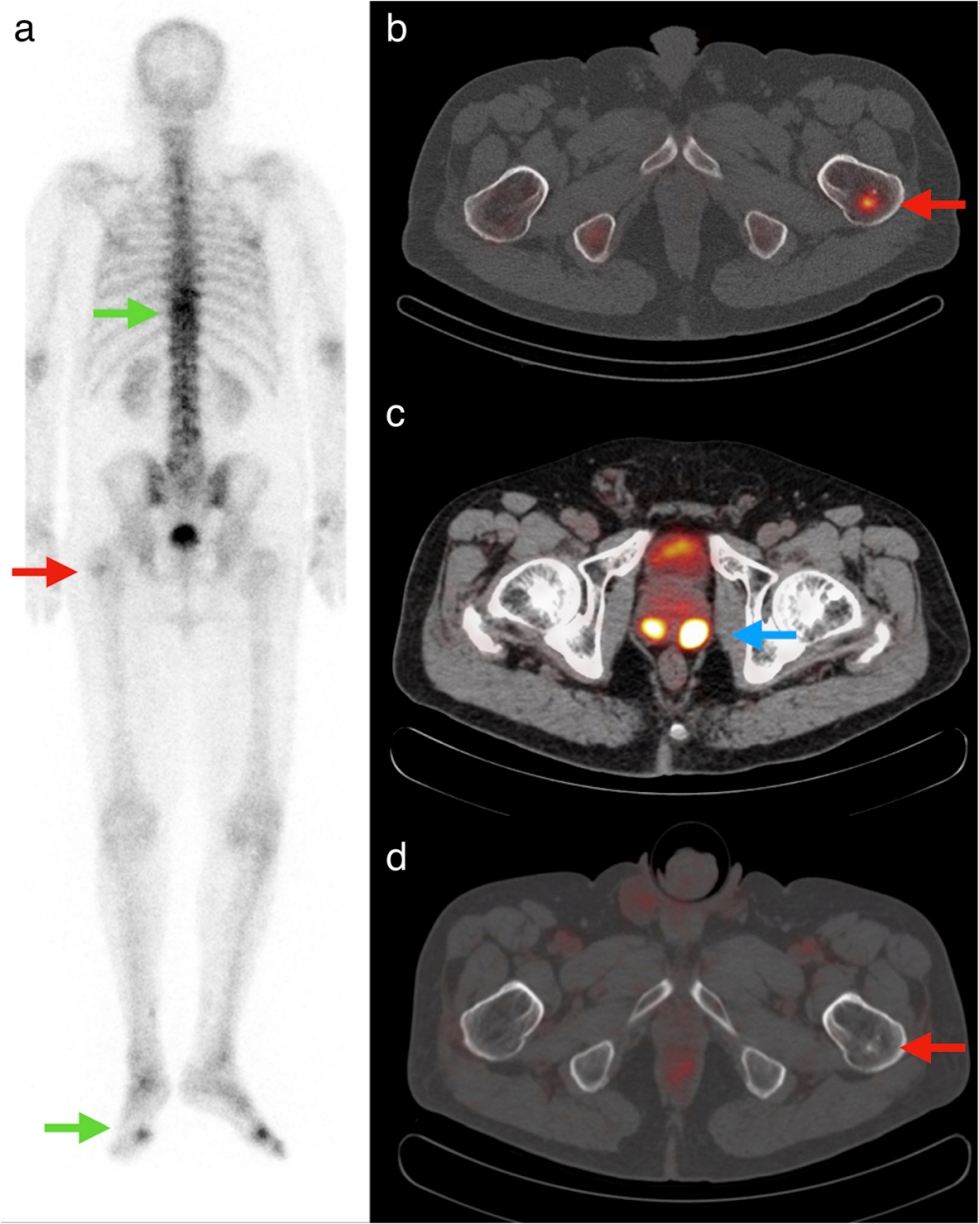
Example of staging change of PSMA PET/CT compared with conventional imaging. A man presented with a clinical T2c Gleason 7b (predominant Gleason score 4) PCa with an iPSA of 16. Planar BS (a) showed a faint spot in the left proximal femur (red arrow) which was confirmed on SPECT/CT (b) as a sclerotic lesion with osteoblastic activity (red arrow). The lesion was interpreted as suspicious for bone metastasis. Other areas with increased osteoblastic activity (green arrows) were interpreted as degenerative. A [^68^Ga]PSMA PET/CT (c) 36 days later shows a PSMA positive bilateral PCa (blue arrow), but no PSMA expression in the sclerotic lesion in the left proximal femur (d, red arrow). Based on PSMA PET/CT the lesion in the left proximal femur was regarded as not suspicious for bone metastasis; M stage changed from positive to negative and treatment intent changed from palliative to curative
